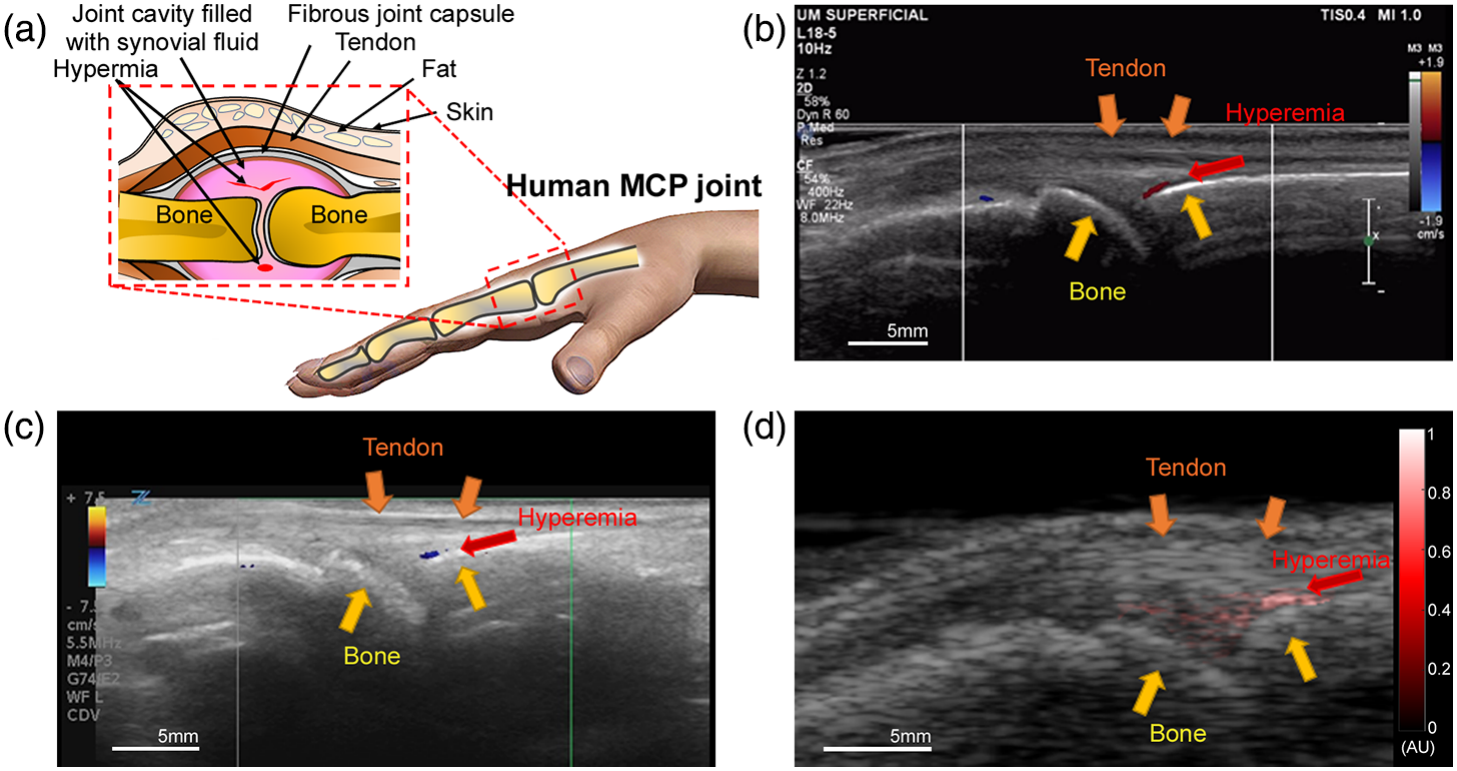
Scanning an inflammatory human MCP joint with US Doppler imaging and LED PAI. (a) Sketch of an inflamed human MCP joint. (b) US Doppler image of the MCP joint obtained during the clinic visit, showing hyperemia in synovium. (c) US Doppler image of the same joint obtained right before PAI scan, indicating hyperemia in the same position. (d) PA and B-mode US combined image of the same joint, where the red pixels in the pseudocolor PA image indicate hyperemia in the same position in the joint.
